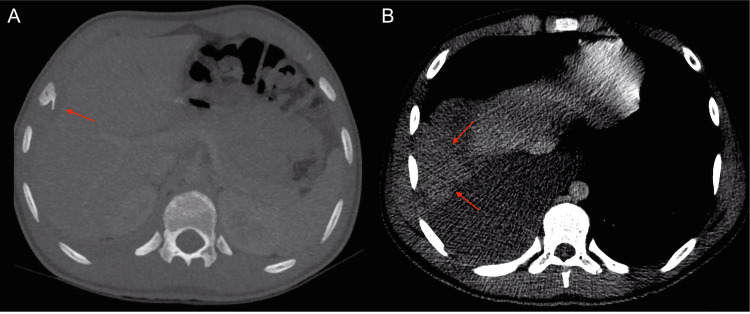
Axial chest CT in bone window (A) revealed an osseous exostosis of the inner aspect of the eighth right costal arch, which contacts and deforms the adjacent hepatic contour (red arrows). In the mediastinal view (B), a high-density pleural effusion (60 HU) was observed (red arrow).
